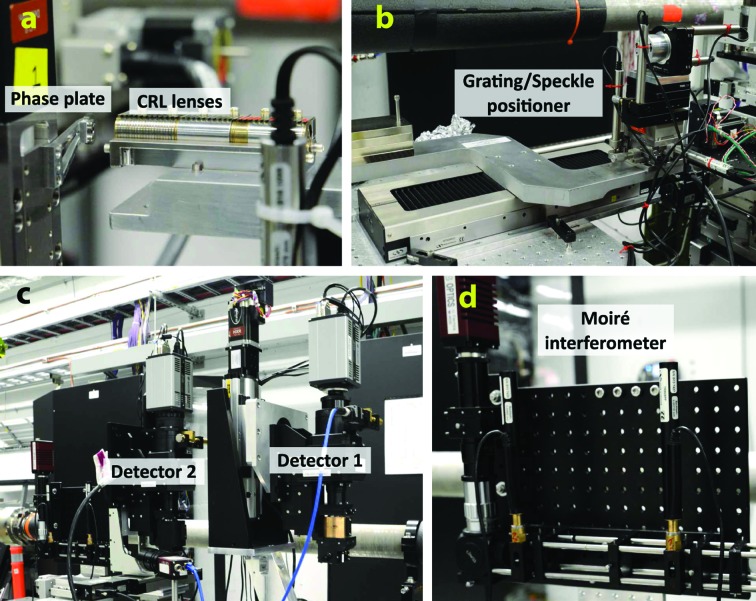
Photographs of the experimental setup. The X-ray beam was focused by a set of beryllium CRL lenses (*a*) and a phase plate with horizontal and vertical degrees of freedom was placed downstream of the CRL optics. The grating/speckle phase modulators were mounted on a motorized positioner with long travel range along the optical axis (*b*). Two indirect X-ray detectors (*c*) with YAG scintillating screens were placed further downstream at approximately 2 m distance from the focus, and the compact moiré interferometer was mounted as the last part of the setup (*d*).
